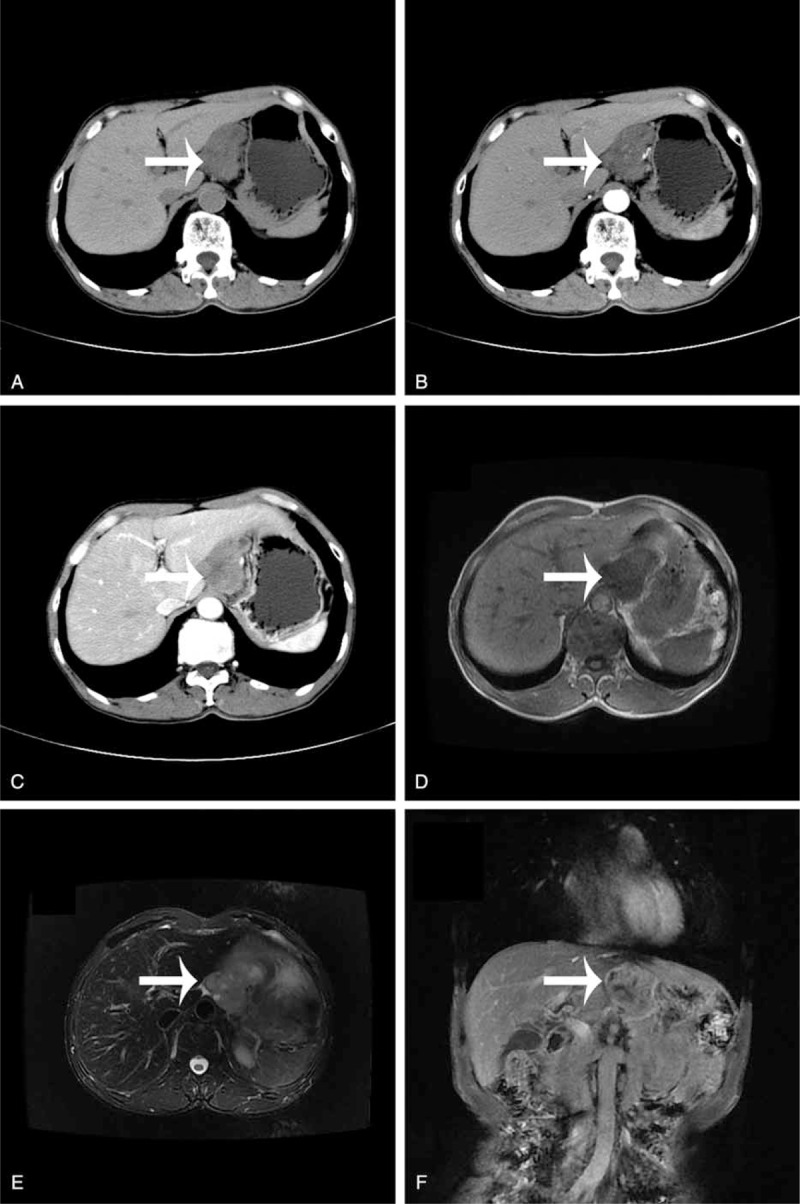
The presence of the carcinosarcoma in the CT and MRI before the peration. A, The CT showed a 5.4 × 4.1 cm^2^ irregular soft tissue mass (white arrow) in the space between the liver and stomach. B, No obvious enhancement (white arrow) was showed in the enhanced abdominal CT scan. C, Slight enhancement (white arrow) was observed in the venous phase. D, The tumor (white arrow) was high signal in T1-weighted MRI. E, T2-weighted imaging revealed no enhancement of the tumor (white arrow). F, The tumor (white arrow) was located in the lesser omentum bursa. CT = computed tomography, MRI = magnetic resonance imaging.
